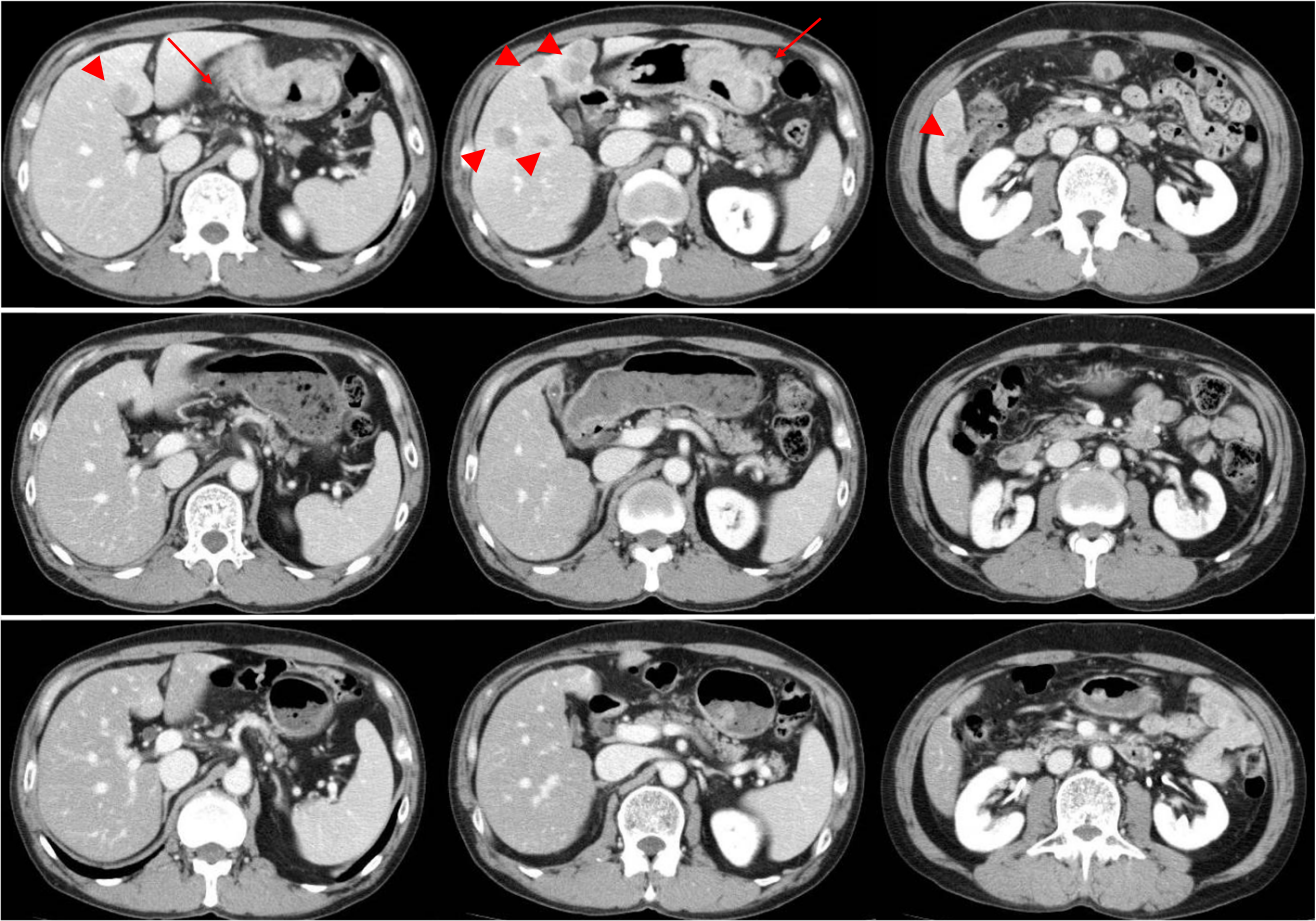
Enhanced computed tomography scan findings. a Thickening of the anterior wall in the *lower* gastric body with enlargement of lymph nodes (*arrow*) along with the lesser curvature and greater curvature. Multiple liver metastases (arrowhead). b, c Complete response of liver metastases and lymph node metastases had been continued
